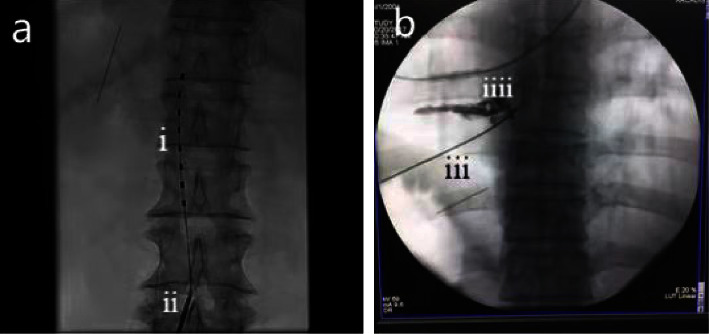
Representative X-ray images taken inside the digital subtraction angiography room; (a) a guided spinal cord stimulation electrode placement in the epidural cavity: (i) spinal cord stimulation electrode and (ii) epidural cavity puncture needle. (b) A guided radiofrequency needle placement in the target nerve, (iii) a radiofrequency needle, and (IV) thoracic spinal nerve under contrast.
